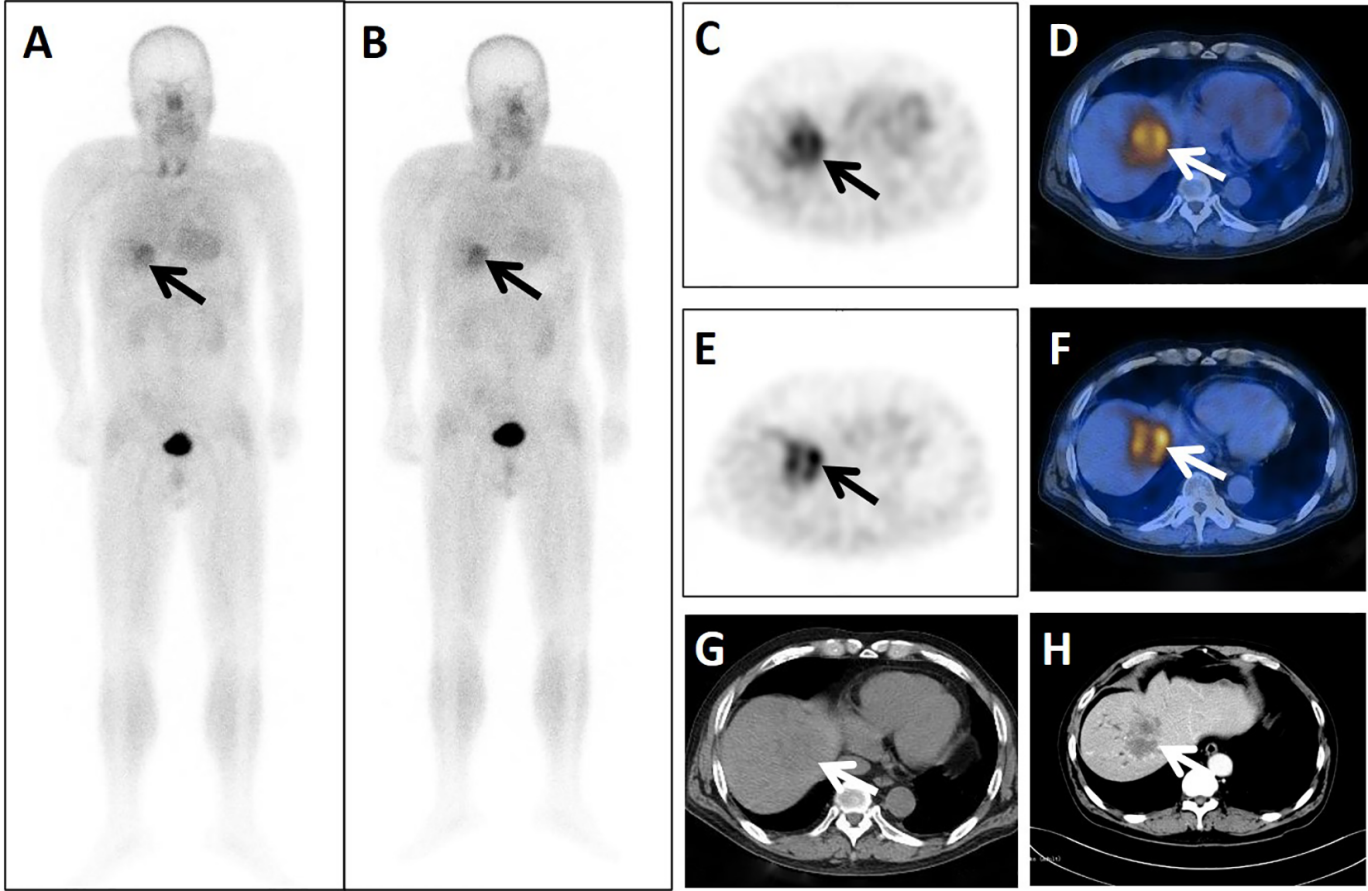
A 24-year-old postoperative male patient with cancer of the transverse colon cancer underwent dual-phase ^99m^Tc-FAPI-04 SPECT/CT. The early anterior whole-body MIP image (A, arrow) showed abnormal accumulation of radioactivity in the liver region. The corresponding axial SPECT image (C, arrow) and fused ^99m^Tc-FAPI-04 SPECT/CT image (D, arrow) of the lesion showed high uptake of ^99m^Tc-FAPI-04 with TBR = 6.38. The lesion was shown more clearly in the delayed anterior whole-body MIP image (B, arrow), corresponding axial SPECT image (E, arrow), and fused ^99m^Tc-FAPI-04 SPECT/CT image (F, arrow) with high uptake and TBR = 6.88. Axial CT image (G, arrow) showed a huge low-density mass measuring 67 mm × 53 mm. The lesion was confirmed to be liver metastasis by enhanced CT (H, arrow), with increased levels of tumor markers CA50 (182.95 ng/mL), CA242 (217.53 IU/mL), and CA199 (2035.75 U/mL).
